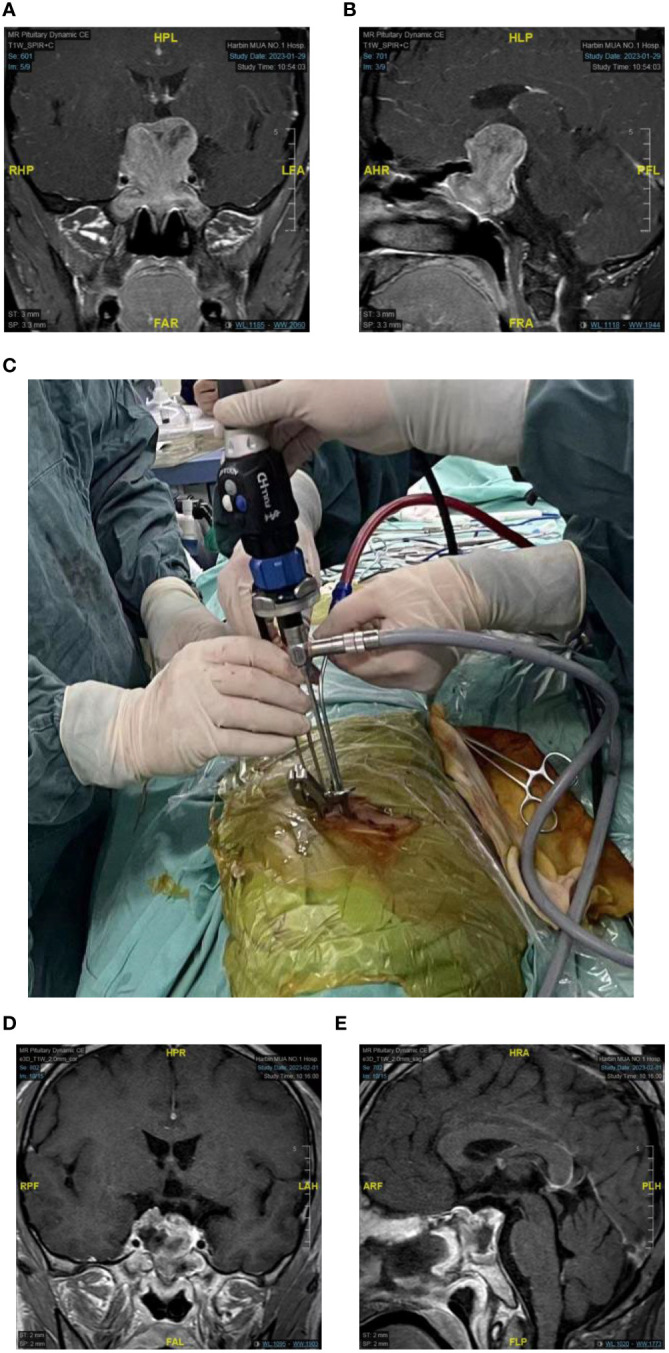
A case of a 32-year-old male presented with progressive visual decline. Preoperatively, the right eye exhibited only light perception, while the left eye had a visual acuity of 0.5, with only a small residual field of vision.The patient was diagnosed with a giant pituitary adenoma (Knosp Grade 3) with bilateral cavernous sinus invasion. (A, B) T1-weighted MRI with gadolinium enhancement [(A) coronal section, (B) sagittal section]. (C) The surgical procedure involved a four-handed, in binasitrol approach with skilled coordination in three surgeons. (D, E) Postoperatively, a significant and immediate improvement in visual acuity and field of vision for both eyes,MRI with gadolinium enhancement: gross total removal of the tumor [(D): coronal sections, (E): sagittal section].
